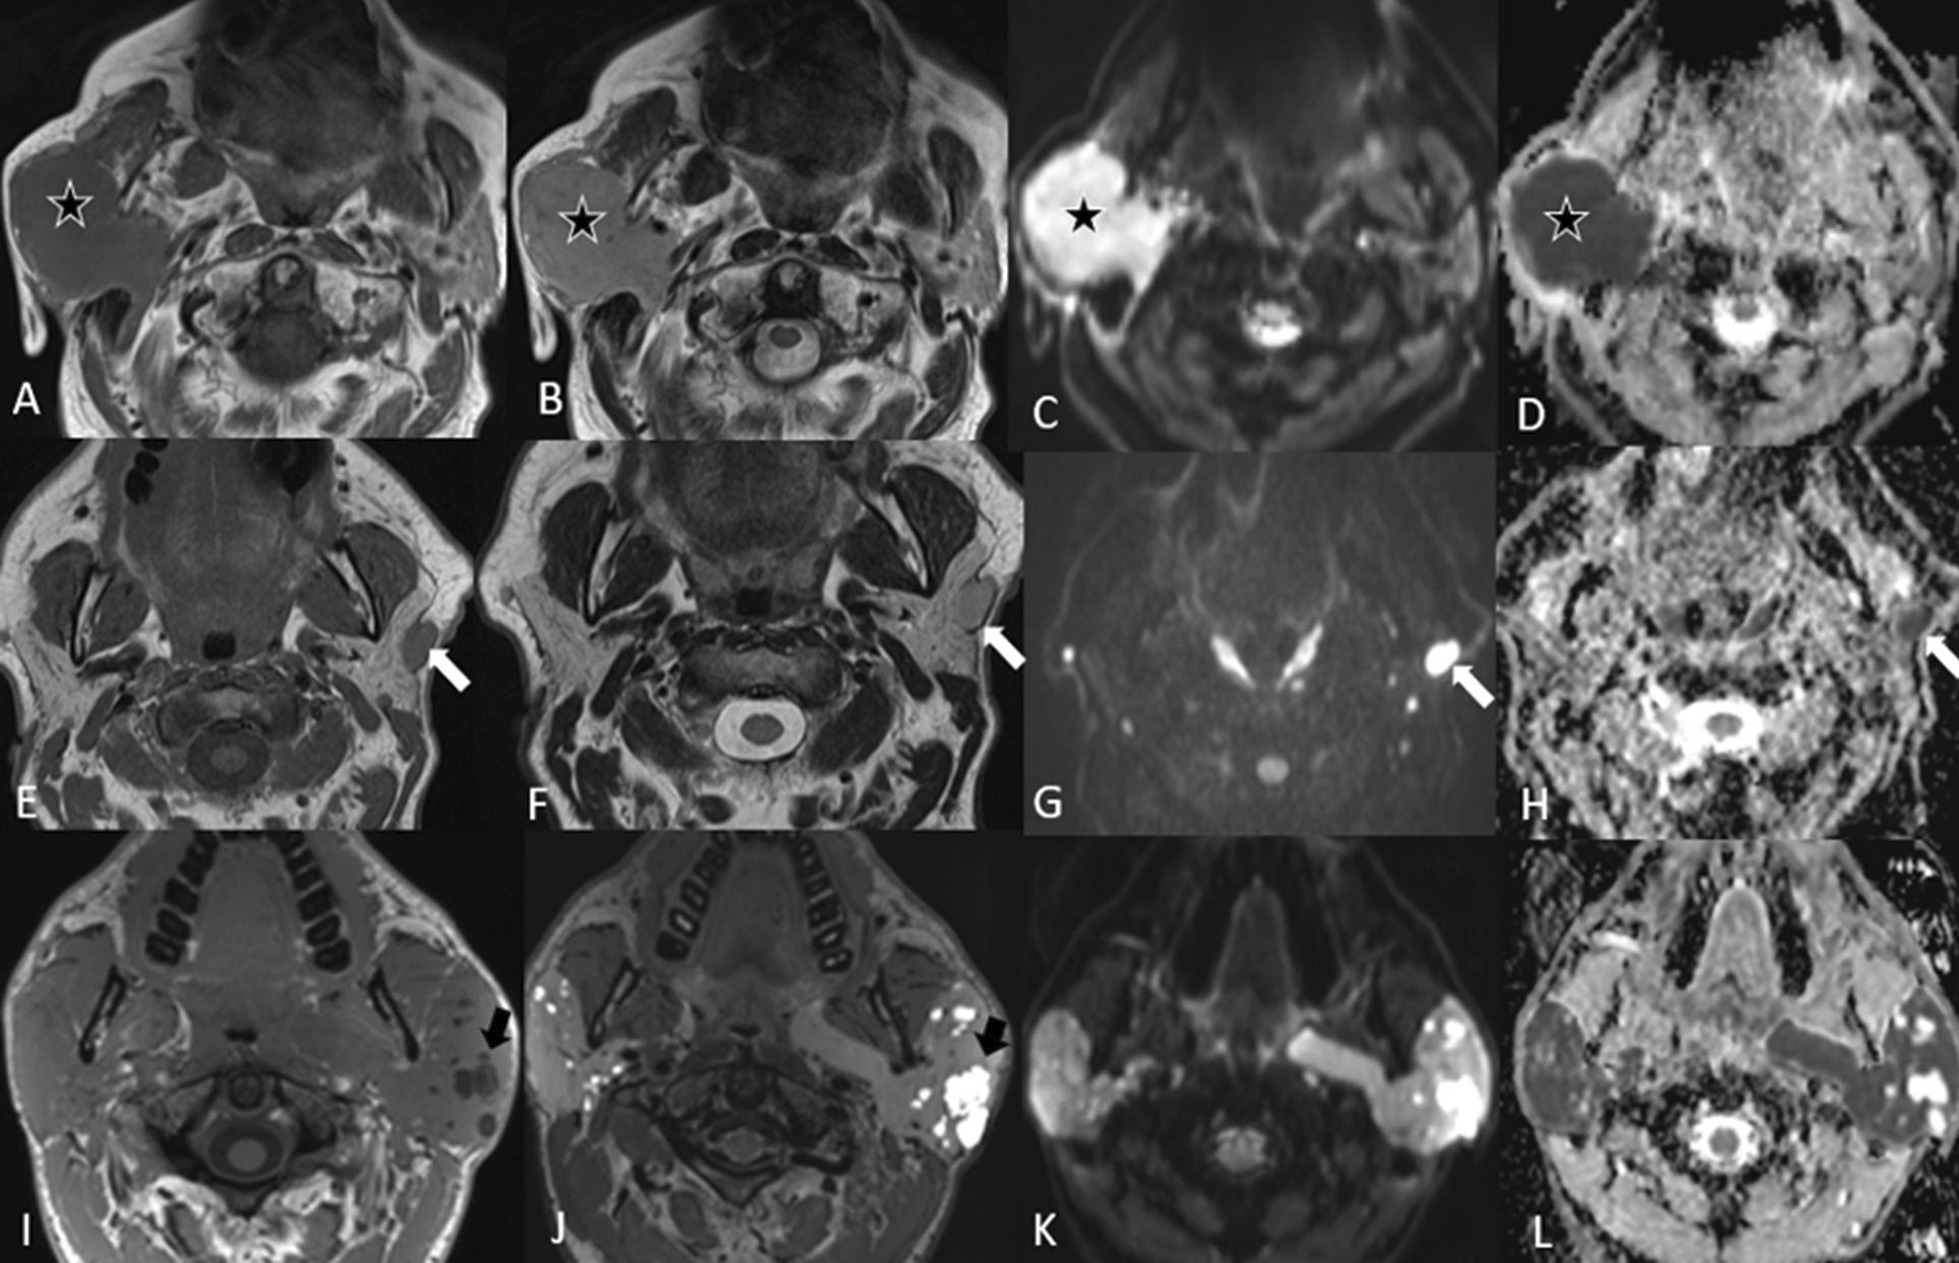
Various radiological appearances of lymphoma: three cases. Patient 1 (A–D) is an 88-year-old male presenting with a swelling in the right parotid gland, which increased in size for 6 weeks. T1-w axial image (A) shows a large, relatively hypointense, homogeneous mass lesion (star) on the right; the lesion is slightly more hyperintense on T2-w (B). The lesion shows very high signal on the DWI (C) with very low signal on the ADC maps (D) revealing the marked diffusion restriction. This was proven by biopsy to be a NHL. Patient 2 (E–H) is a 66-year-old male with an unclear swelling in the left parotid gland. There were two nodules in the parotid gland on the left. The T1-w image (E) shows one lesion (arrow) which is hypointense to the parotid gland and isointense to the skeletal muscles on T1-w, the lesion appears more hyperintense on T2-w images (F) but it is still slightly hypointense to the parotid gland. Furthermore, the diffusion-weighted image (G) and ADC map (H) show marked diffusion restriction. This was a case of follicular NHL. Patient 3 (I–L) is a 36-year-old male with Sjögren’s syndrome. Axial T1-w (I) and axial T2-w (J) images show the diffuse enlargement of the parotid glands with multiple small cysts (black arrow). The DWI (K) shows some hyperintensity in the parotid gland with a low signal in the ADC map (L) indicating diffusion restriction. This was proven to be extranodal marginal zone lymphoma of the parotid gland
